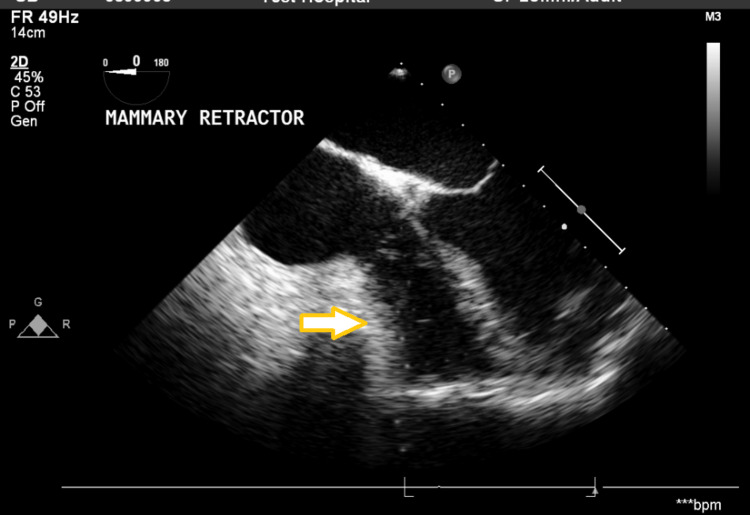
Transoesophageal echo in the four chamber view showing compression of the Right Ventricle by the mammary retractor (arrow).
